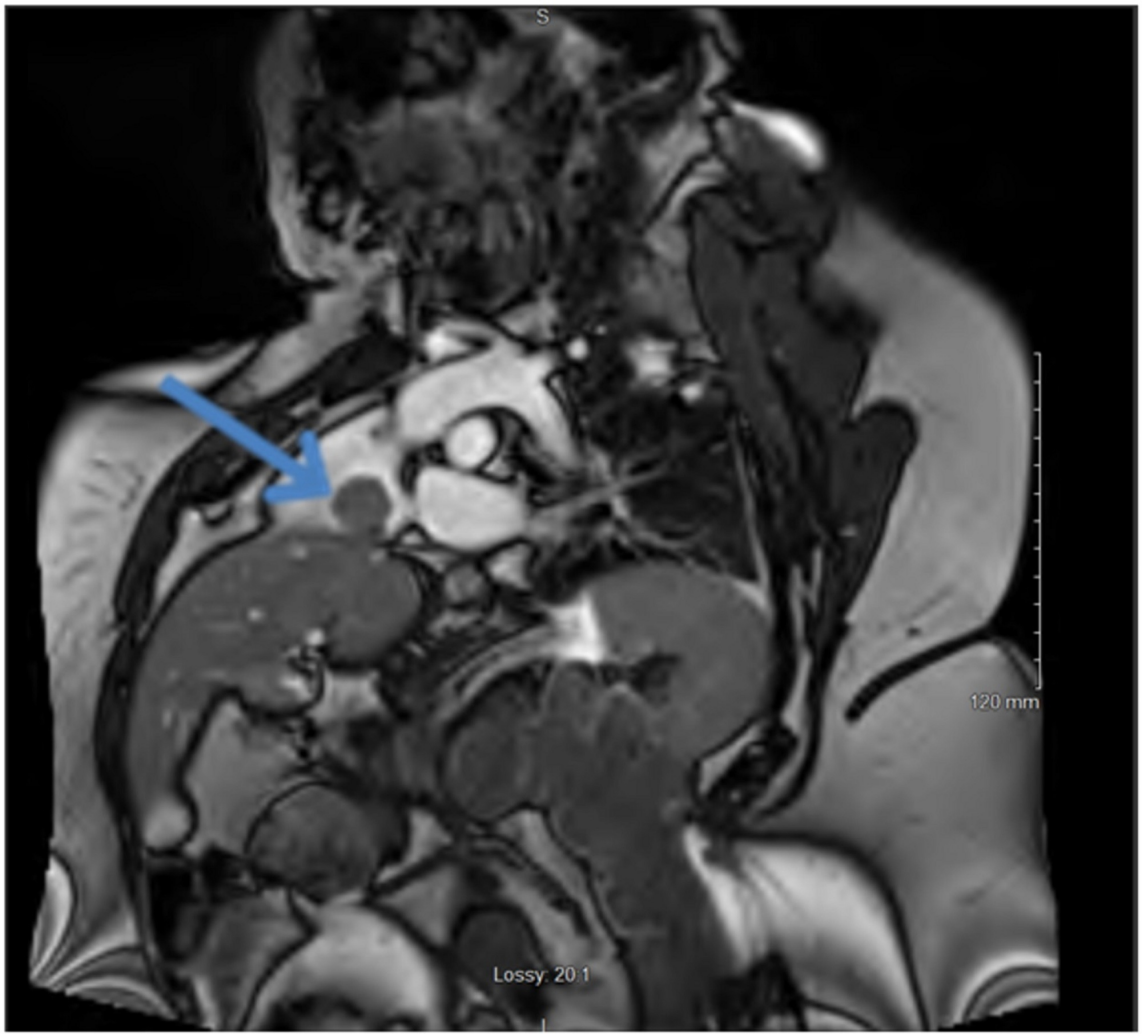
Cardiac magnetic resonance imaging (MRI) MRI image of cine sequence cutting through a right atrial mass showing the origin of the mass from the liver
